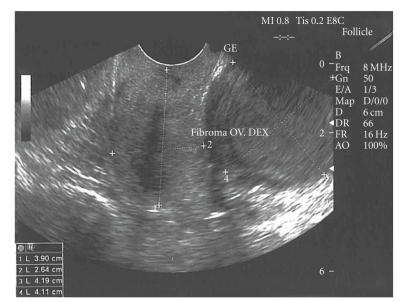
Intravaginal ultrasonography shows a 3.9 mm cyst mass in the right lower abdominal quadrant.
